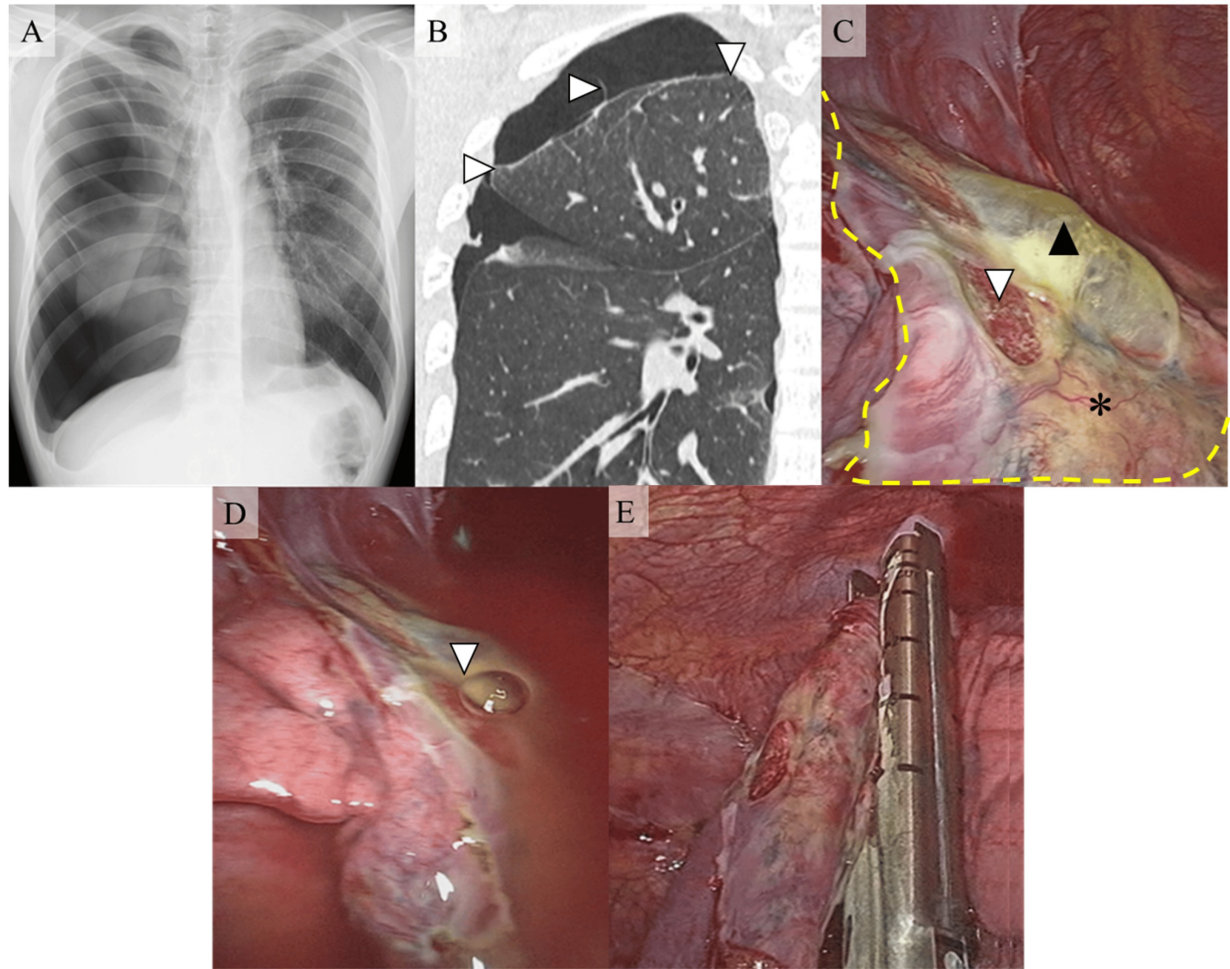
Radiological images and intraoperative findings of the second surgical treatment A: Chest X-ray revealed complete right-side spontaneous pneumothorax; B: Adhesion between the right upper lobe and chest wall (arrow) was found on chest computed tomography, and no obvious overlooked or regrown bullae were found; C: Lung laceration (white arrow) was found in the area without adhesion (*) next to the released adhesion (yellow line). A part of an ablated bulla or degenerated ORC mesh sheet was found nearby (black arrow); D: The air from the laceration (arrow) was found in the water-sealing test; E: The laceration was resected with an endo stapler. ORC: Oxidized regenerated cellulose
